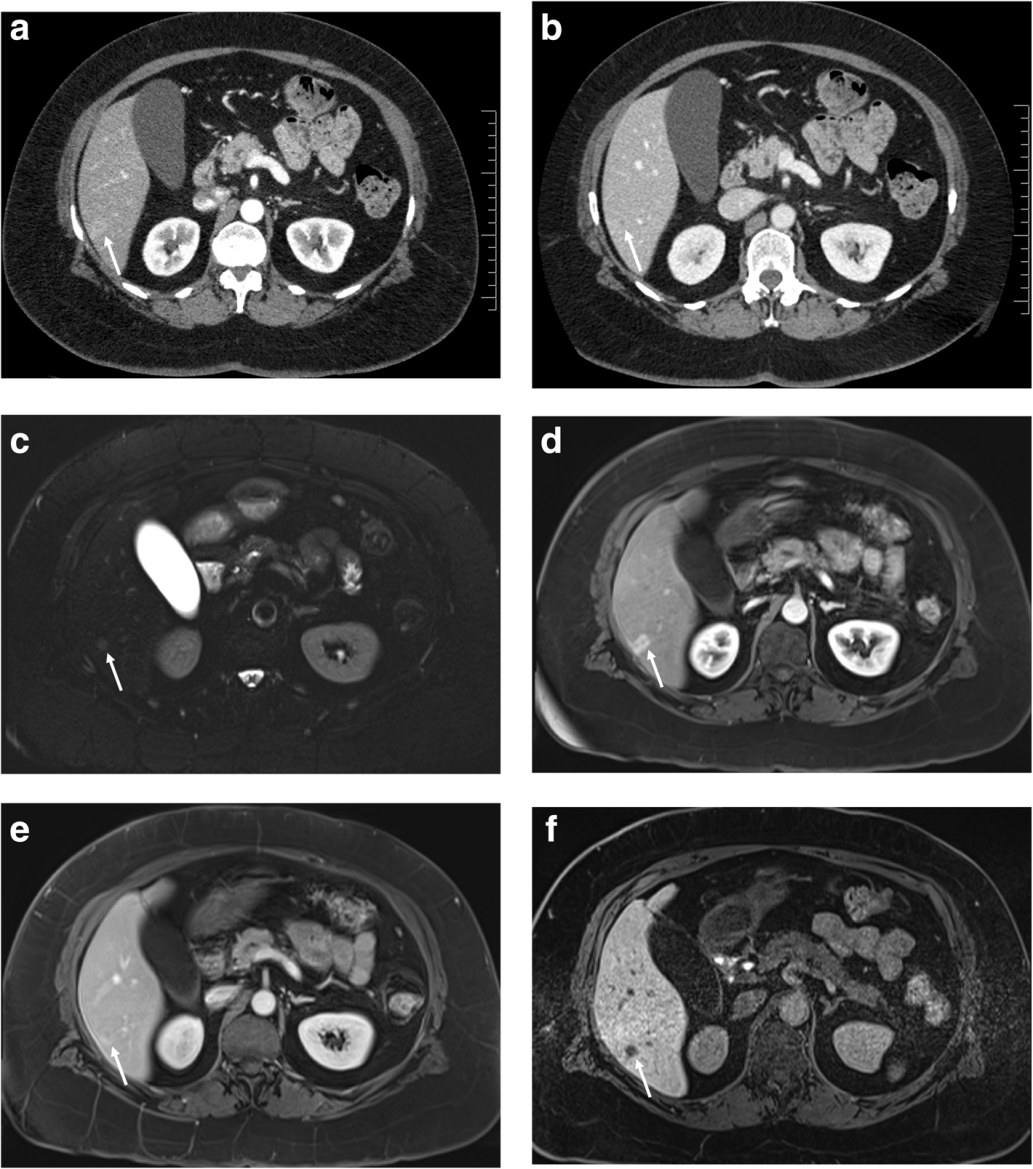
A 55-year-old female with pancreatic cancer and indeterminate lesion on CECT but diagnosis of liver metastasis on EOB-MRI (arrows). There is a vascular blush in segment 6 on arterial phase of CT scan (a), measuring 9 mm. On the portal phase of CT scan (b), a subtle, indeterminate low-attenuation lesion is evident. On EOB-MRI, a mildly T2 hyperintense nodule (c) demonstrates peripheral enhancement with central hypoenhancement in arterial (d) and portal (e) phases and marked hypointensity on the hepatobiliary phase (f). The patient was excluded from surgery, and the percutaneous focal liver lesion biopsy confirmed metastatic adenocarcinoma
